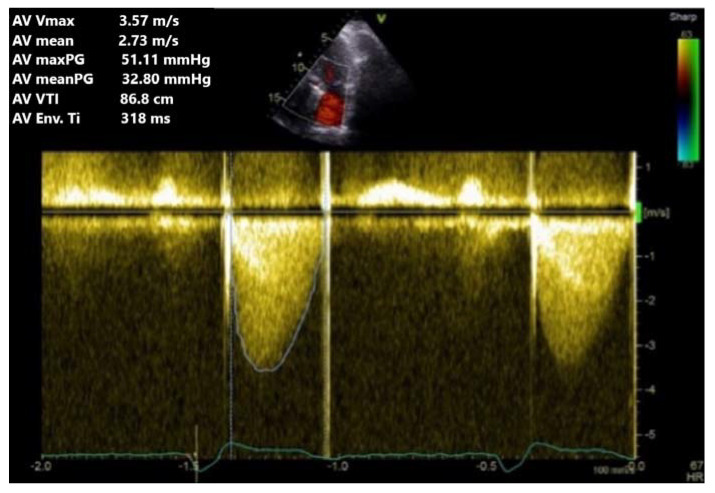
TTE CW Doppler. Obstruction of aortic prosthesis with moderate maximum and medium gradients and velocity.
